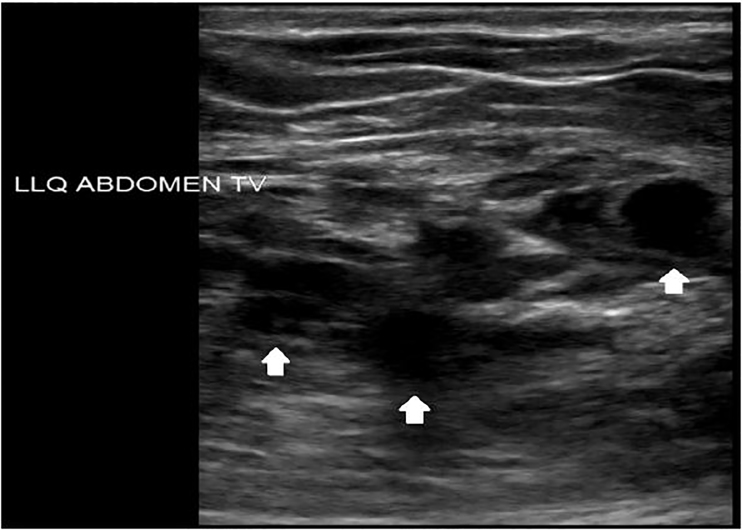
Transabdominal ultrasound of the mass in axial plane demonstrating anechoic, well-circumscribed structures (arrows).
